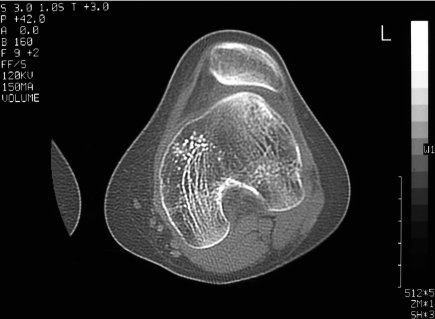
Preoperative computed tomography demonstrating marked trochleodysplasia of the femur, with a sulcus angle of 170°.
